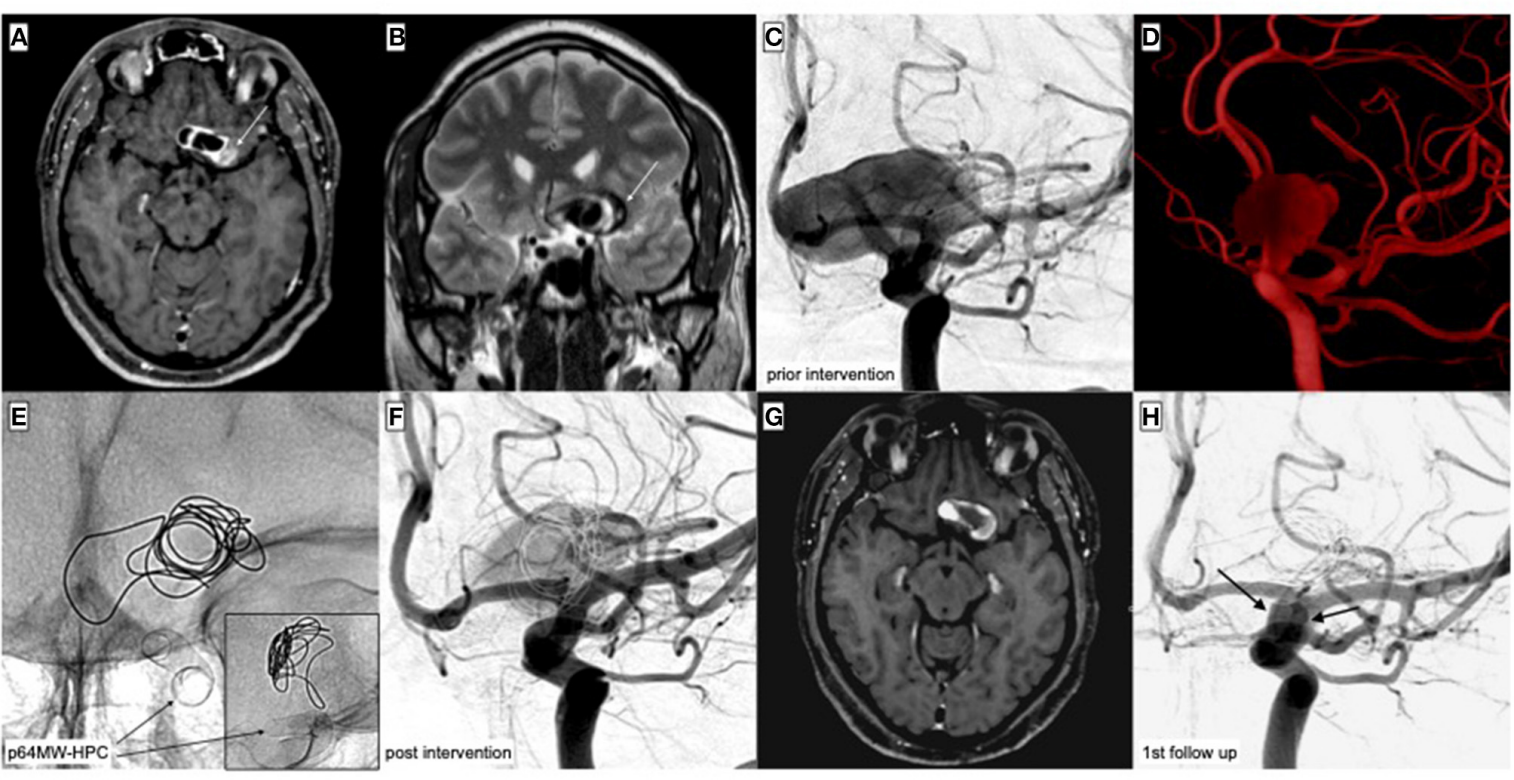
Treatment of a partially thrombosed, giant aneurysm of the C6 segment of the left-handed side ICA. The aneurysm had compressed the ipsilateral optic nerve and caused unilateral blindness. The cause for unilateral blindness had remained unclear for months—finally, MR imaging was performed and demonstrated the underlying pathology. The decision was made for flow diversion together with loose additional coiling, in order to further decrease intra-aneurysmal flow. (A) Contrast-enhanced, axial T1-weighted imaging shows the partially thrombosed (white arrow) giant aneurysm of the left-handed side ICA. (B) Coronal T2-weighted imaging demonstrates the multi-compartmental (white arrow) aneurysm and its significant space-occupying effect. (C) DSA in posterior–anterior projection shows the 26 × 17 mm perfused compartment of the aneurysm. (D) Reconstruction of the 3D rotational angiogram demonstrating the neck level of the giant aneurysm. (E) Radiography in posterior–anterior projection post implantation and coiling. The distal end of the p64MW-HPC was positioned in the C7 segment of the ICA, avoiding affection of the ICA-T. One coil was placed inside the aneurysm in order to further disrupt intra-aneurysmal flow. The image in the lower right corner displays the lateral projection after FDS implantation and supplementary coiling. (F) DSA immediately after implantation of the FDS. Note the already decreased volume of the residually perfused compartment. Compared to (C), aneurysmal perfusion is markedly delayed. (G) Contrast-enhanced, axial T1-weighted imaging 5 weeks post procedure demonstrating the significantly decreased volume of the aneurysm together with progressive thrombosis of the peripheral compartments. (H) First angiographic follow-up in posterior–anterior projection 3 months post intervention. The aneurysm is sufficiently separated from the parent vessel (OKM C2).
